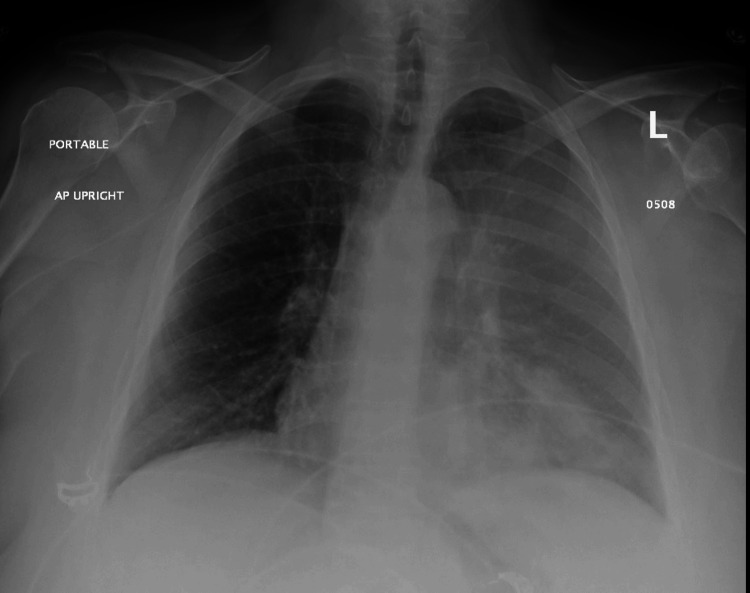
Chest X-ray at the time of presentation with pulmonary symptoms showing patchy infiltrates
